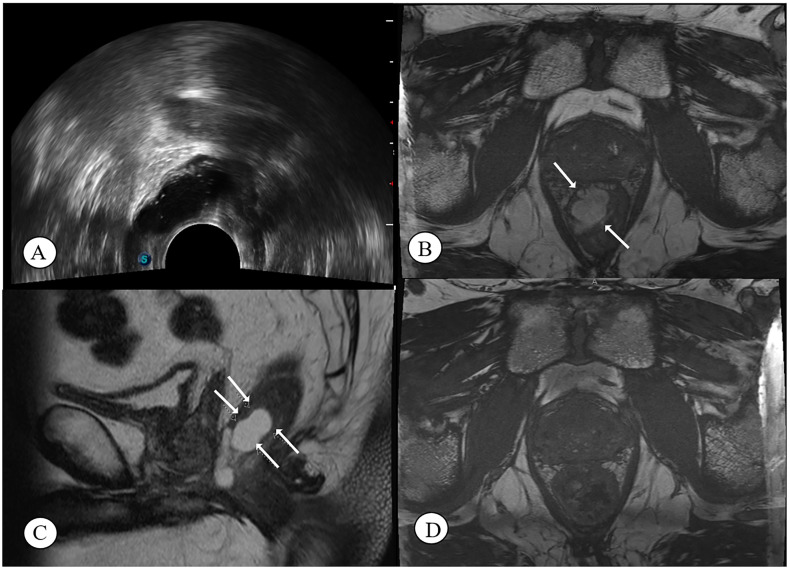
(A) Ultrasound imaging at the time of the procedure. (B, C) Diagnostic MRI showing rectal wall infiltration (white arrows). (D) Treatment planning MRI showing resolution of rectal wall infiltration after hyaluronidase injection.
